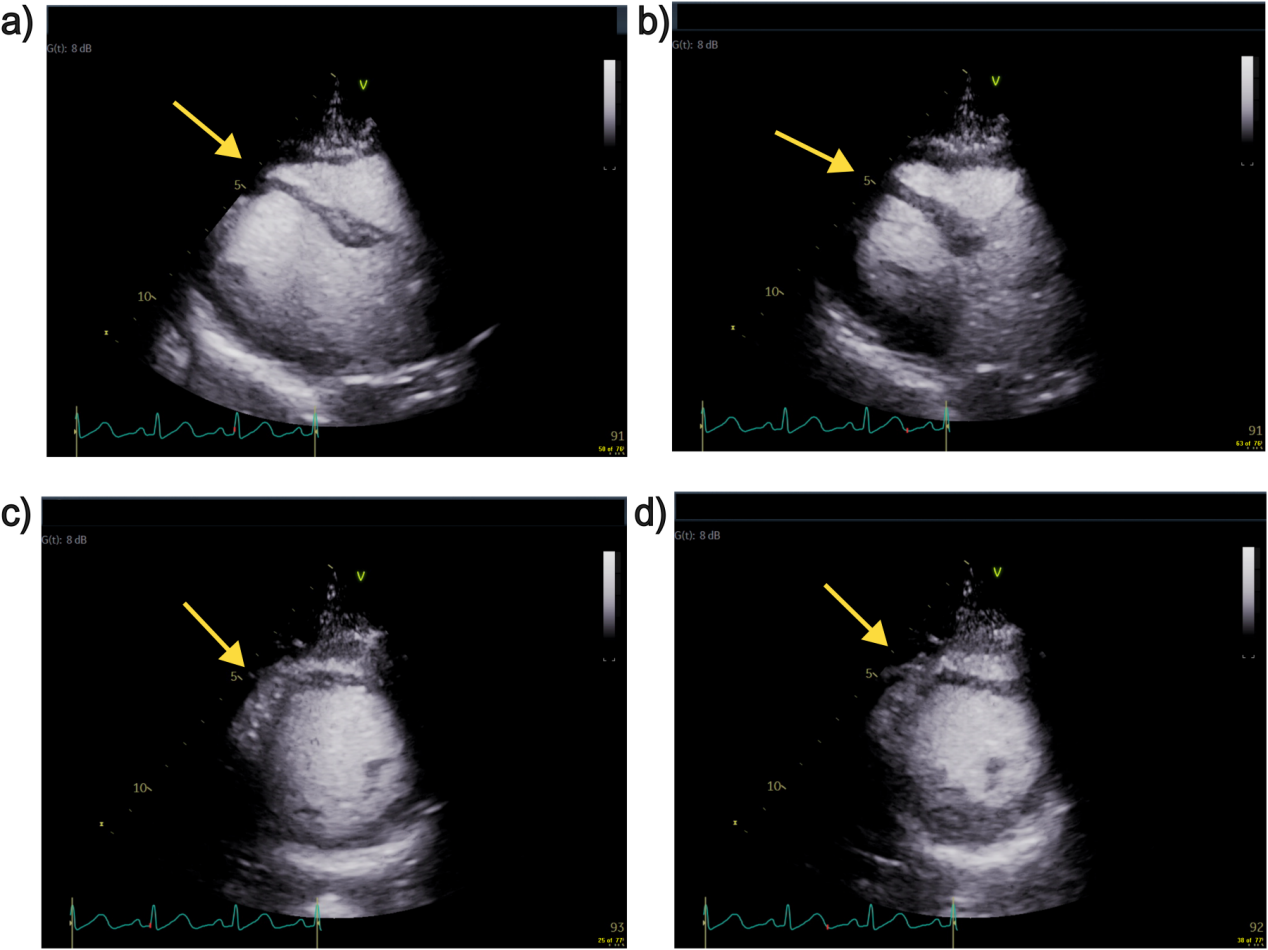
TTE images extracted as freeze frames. (a) Parasternal long axis view of end diastole. (b) Parasternal long axis view of end systole. (c) Parasternal short axis view of end diastole. (d) Parasternal short axis view of end systole. Yellow arrows indicate site of hypokinesis.
